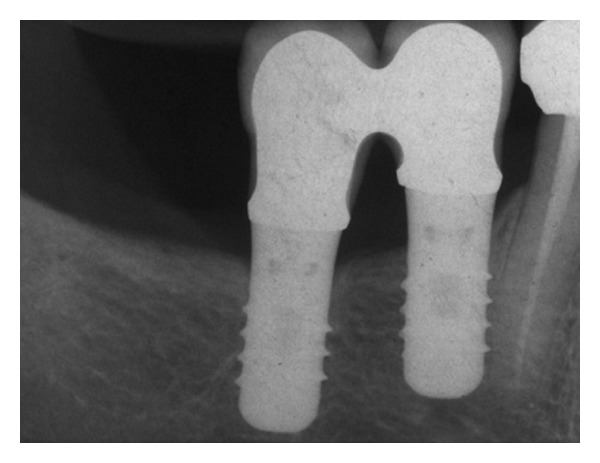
Partial fixed prosthesis supported by short implants (4.1 × 8 mm), 0 years loading. Periapical radiograph.
